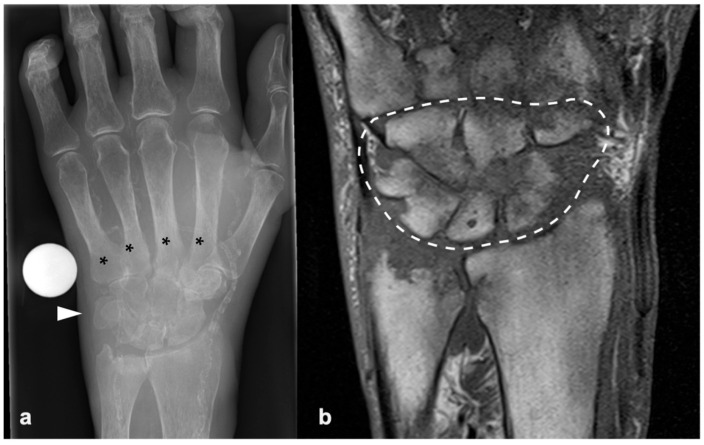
Preoperative imaging. (a) X-ray of the wrist with extensive bone destruction, affecting the whole carpus (arrowhead) and metacarpal bases (asterisks). (b) Magnetic resonance imaging of the wrist, highlighting arthritis and carpal bone osteomyelitis (dotted line).
